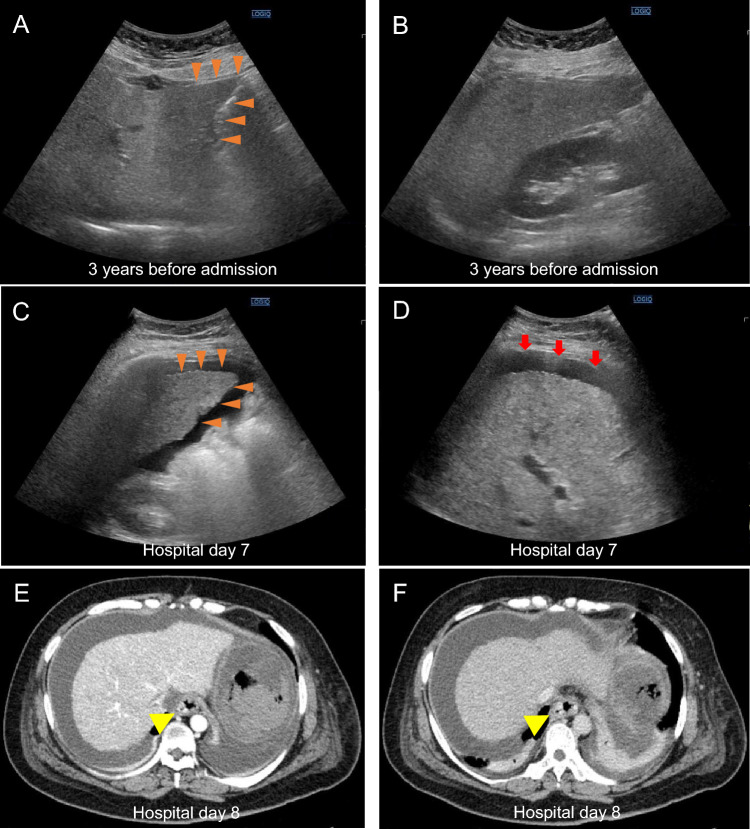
Abdominal ultrasonography and contrast-enhanced computed tomography findings. A, B Ultrasonography three years before admission: A Blunted liver edge with an irregular surface (arrowheads). B Hepatorenal contrast showing increased hepatic echogenicity, consistent with hepatic steatosis. C, D Ultrasonography at referral (hospital day 7): C Irregular liver surface (arrowheads) with coarse parenchymal echotexture. D Moderate ascites (arrows) without evidence of hepatic malignancy. E, F Contrast-enhanced CT on hospital day 8: Dilated vessels in the lower esophagus (arrowheads) without active bleeding
